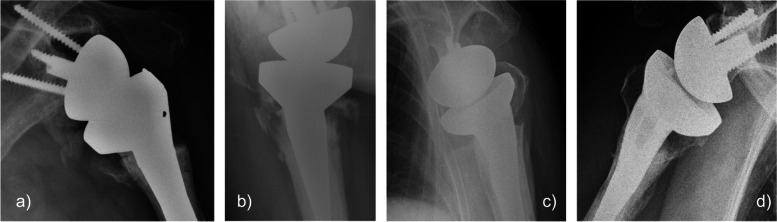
Radiographic evaluation of tuberosity healing. Four different classifications: a resorption; b non-union; c malunion; and d anatomical healing
